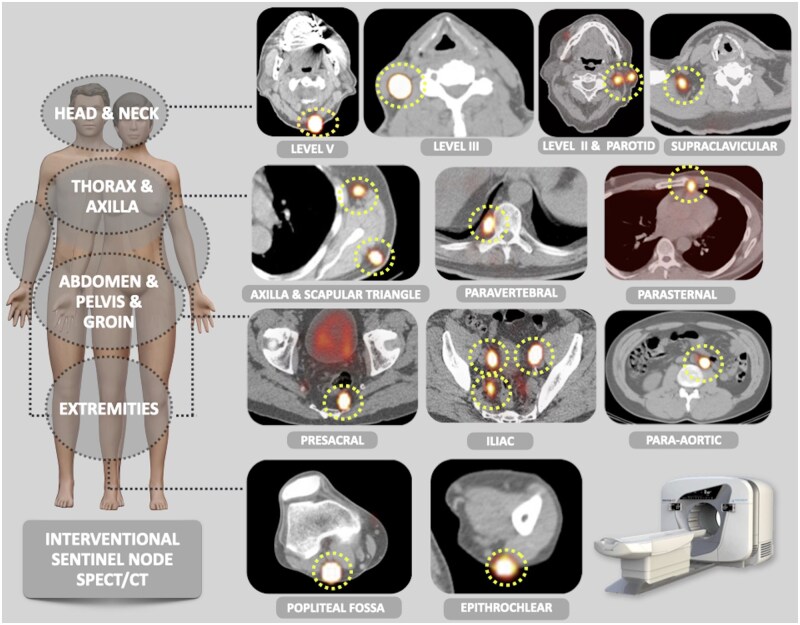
Interventional SPECT/CT overview showing examples of anatomical sentinel node localization in diverse parts of the body (circles).
